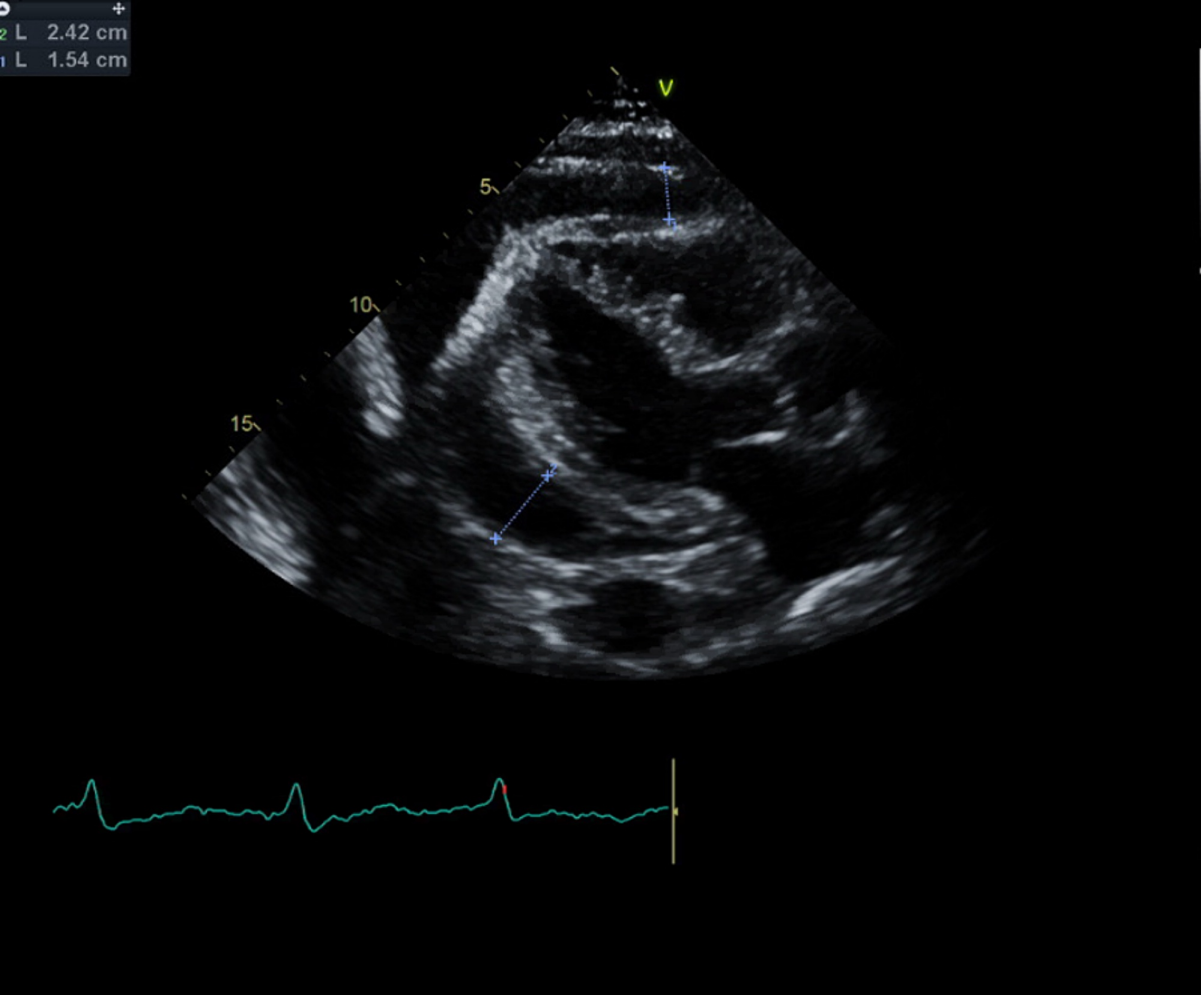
Parasternal long-axis view on echocardiogram remarkable for a large pericardial effusion.
